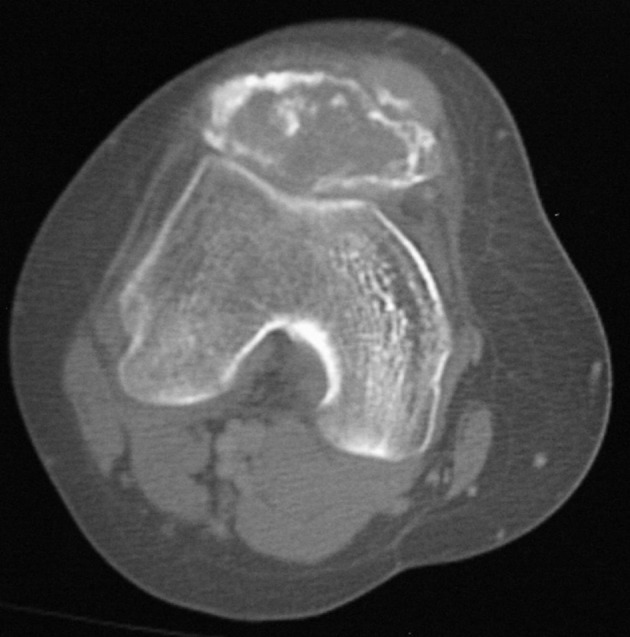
Computed tomography showing an intraosseous osteolytic lesion and soft tissue extension at the anterior part to the patella.
